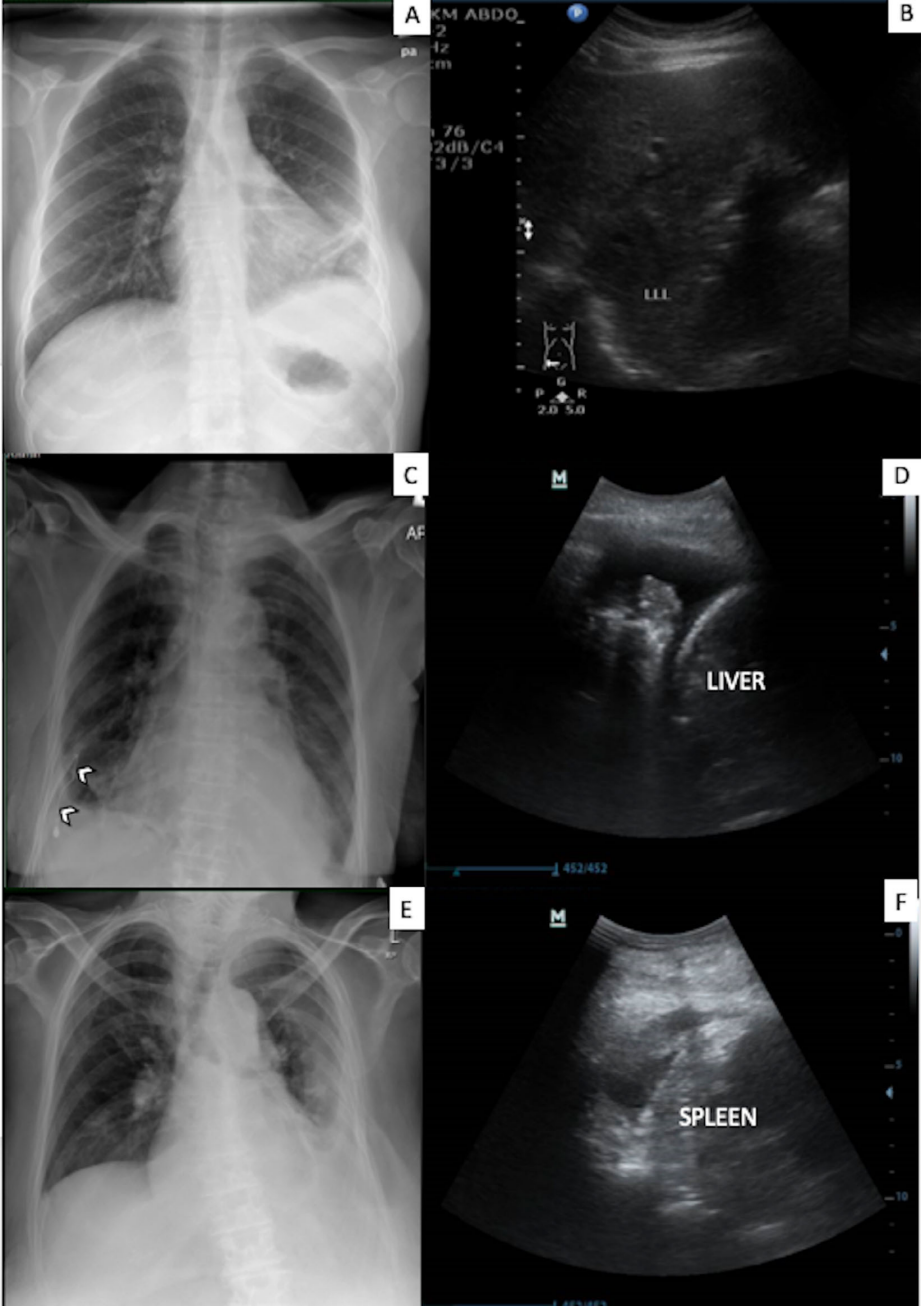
Imaging post three doses of intrapleural fibrinolysis (alteplase and deoxyribonuclease (DNase)). Patient 1: Chest radiograph (A) showed minimal left pleural effusion. Ultrasound (B) on follow‐up showed resolved liver abscess and pleural effusion. Patient 2: Chest radiograph (C) showed minimal right pleural effusion and chest catheter in situ (white arrows). Ultrasound (D) revealed unilocular effusion (compared to septated effusion before therapy). Patient 3: Chest radiograph (E) and ultrasound (F) showed minimal left effusion.
